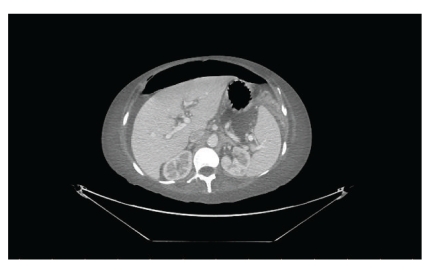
CT scan showing free air in the abdomen.
